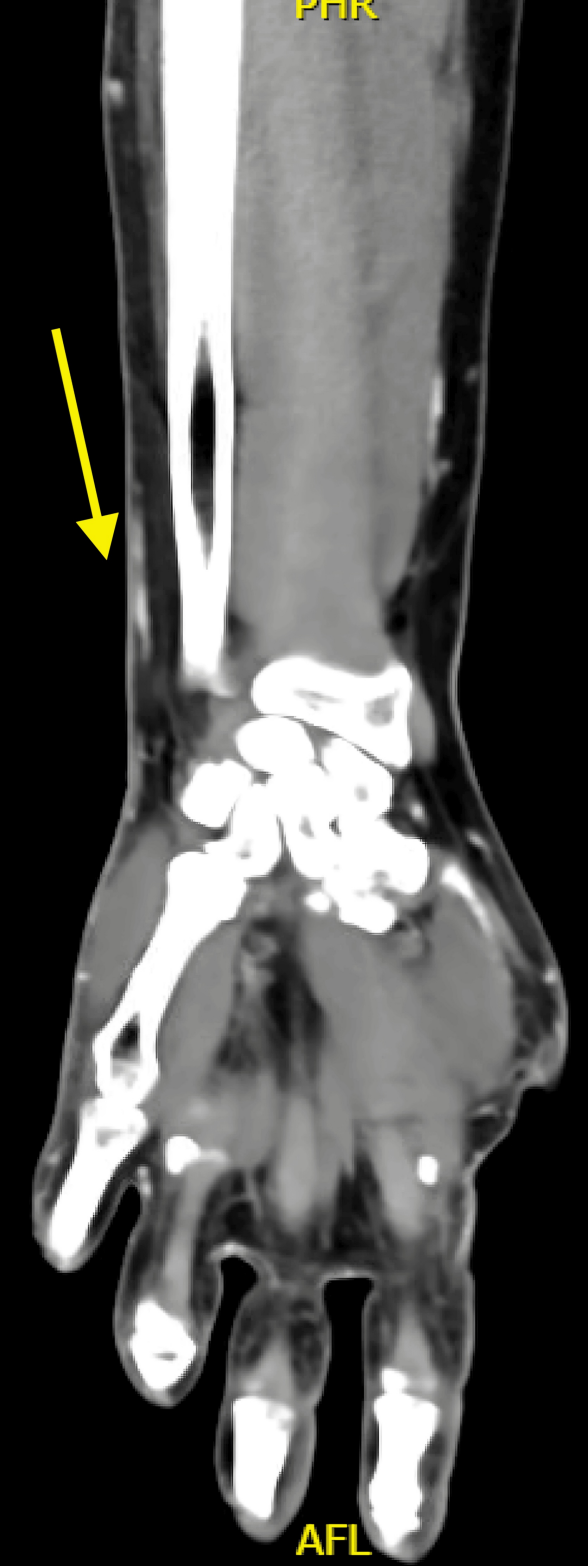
CT scan of upper extremity, coronal view The arrow points to fat stranding, which is concerning for soft tissue infection. AFL: Anterior forearm left-sided
